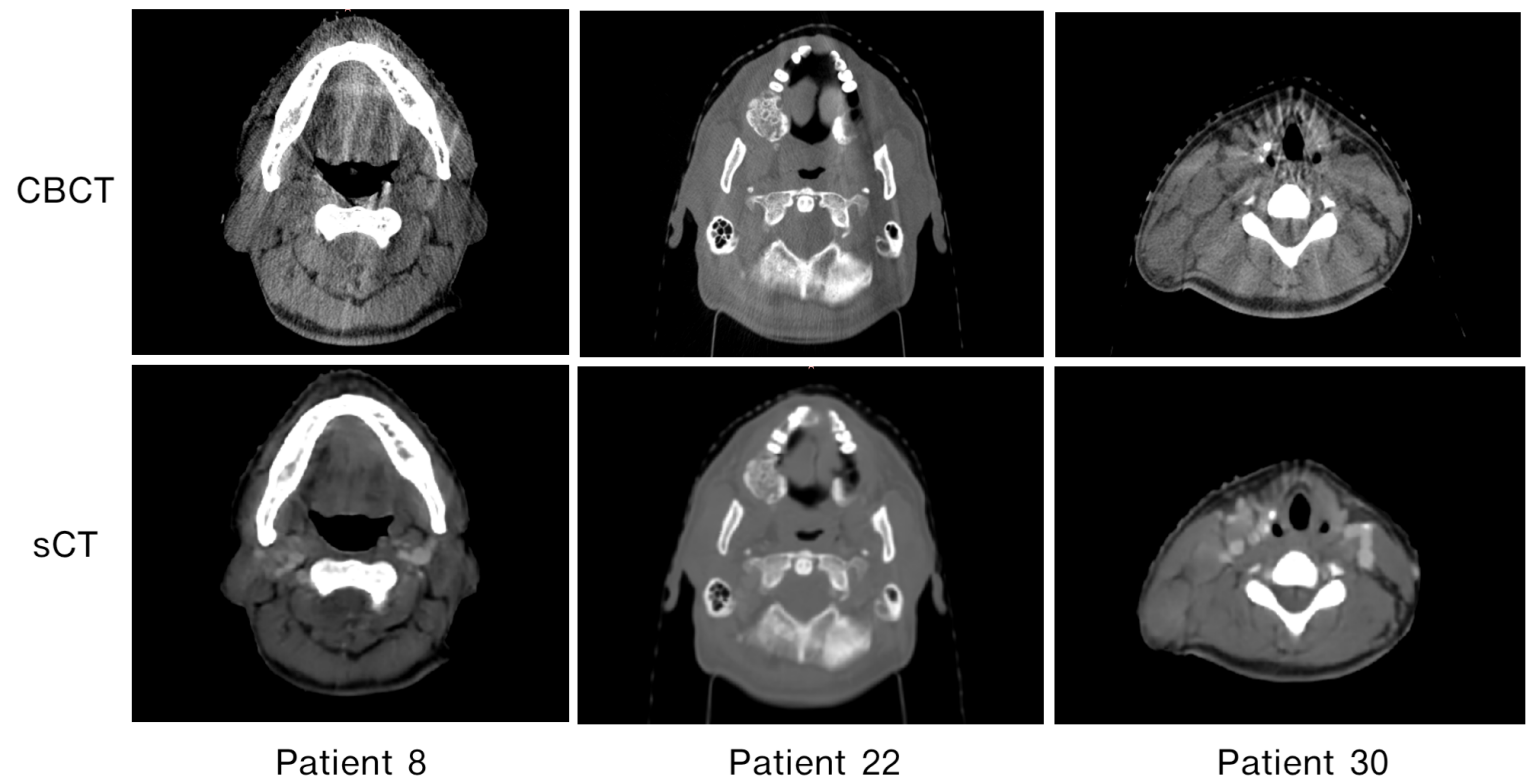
Comparison of CBCT and sCT on axial slices of patients with the three highest average MAEs (Patients 8, 22, and 30).
